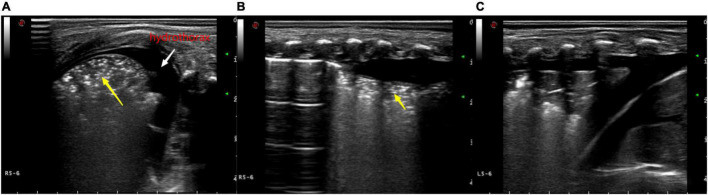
Ultrasonic manifestations of neonatal pulmonary consolidation combined with pleural effusion. Pulmonary ultrasound shows consolidation area with air bronchogram (yellow arrow). A-line disappears, and pleural effusion can be observed. (A) Longitudinal image of the intercostals. (B,C) Transverse image along the intercostals.
